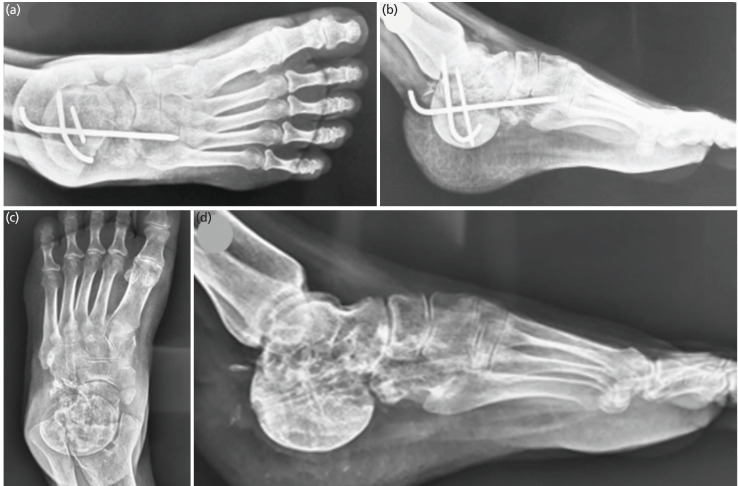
(a and b) Follow-up radiographs at three months showing early radiological signs of graft incorporation. (c and d) The 36 months follow-up radiographs (AP lateral radiographs), showing complete incorporation of allograft, no local recurrence and resorption, with some alteration in calcaneal pitch angle.
